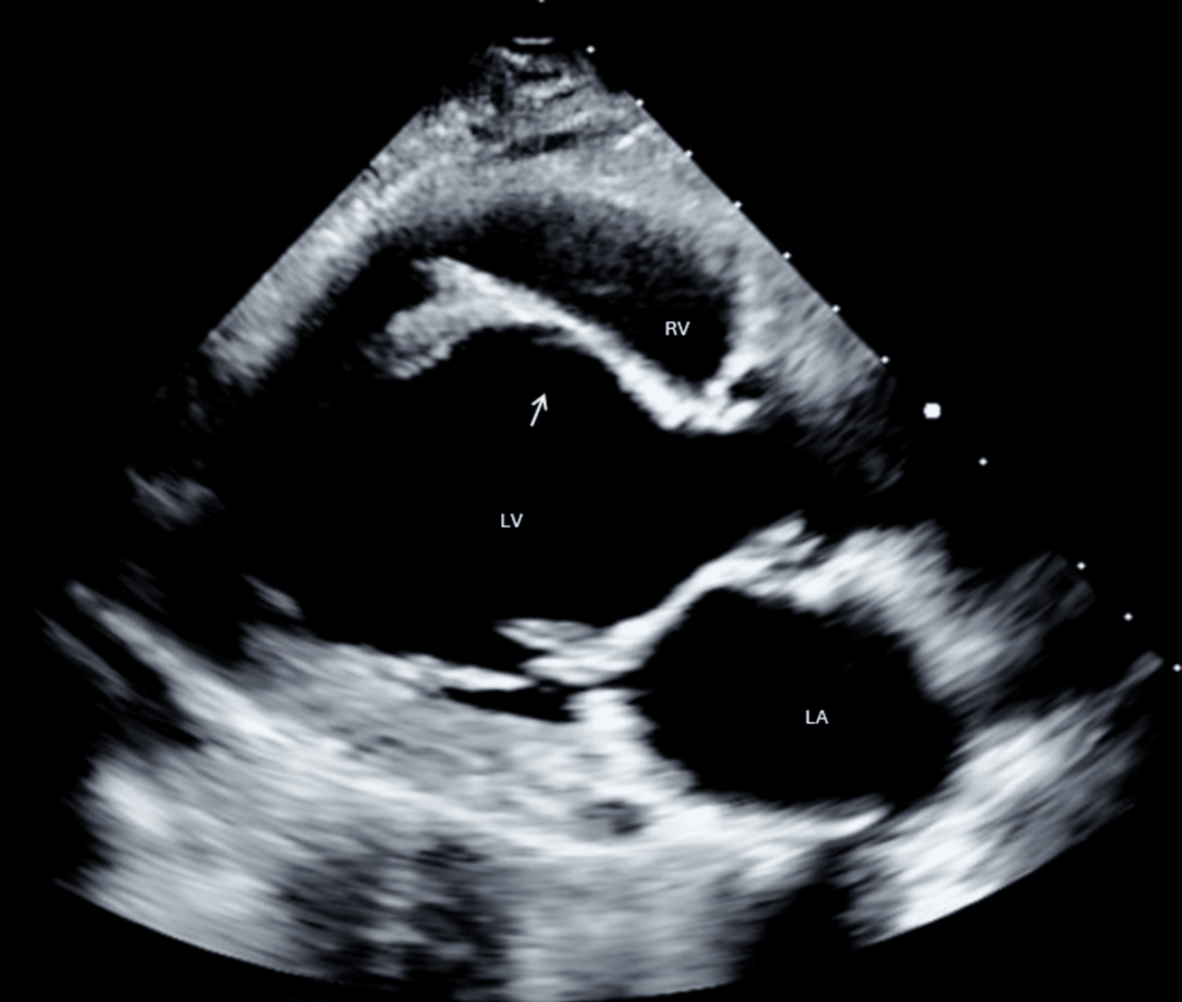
TTE completed approximately four months prior to onset of symptoms. Arrow points to septal aneurysm with bowing into right ventricle during systole. TTE: Transthoracic echocardiogram
